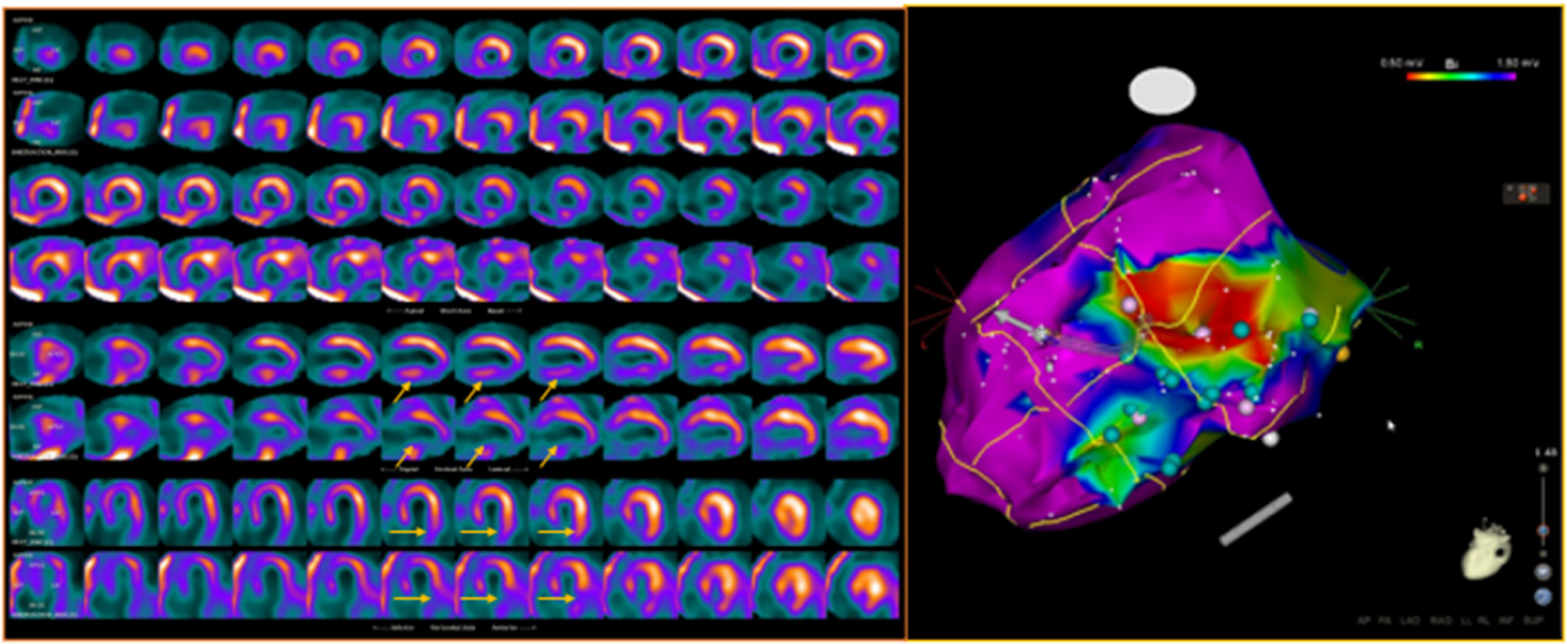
Dual-tracer scintigraphy in a patient with previous inferior myocardial infarction and recent episodes of ventricular tachycardia. Left: The ^99m^Tc-tetrofosmin rest single-photon emission CT (SPECT) images show the presence of an inferior myocardial infarction (top rows; SRS 12). The ^123I^-MIBG (bottom rows) innervation images showed a large area of absence of tracer uptake (no-innervation) from the basal to mid-ventricular lateral walls and in the inferior wall (SS-MIBG 19). A large area of innervation/perfusion mismatch was detected in the lateral left ventricular (LV) wall. Right: The electrophysiology (EP) study identified the arrhythmic focus in the inferior and infero-lateral LV walls, corresponding to the area of denervated myocardium. The ablation procedure was performed at the level of the inferior scar as well as of the viable infero-lateral wall. Pink dot, fragmented signals. White dot, late potential. Blue dot, double potential.
